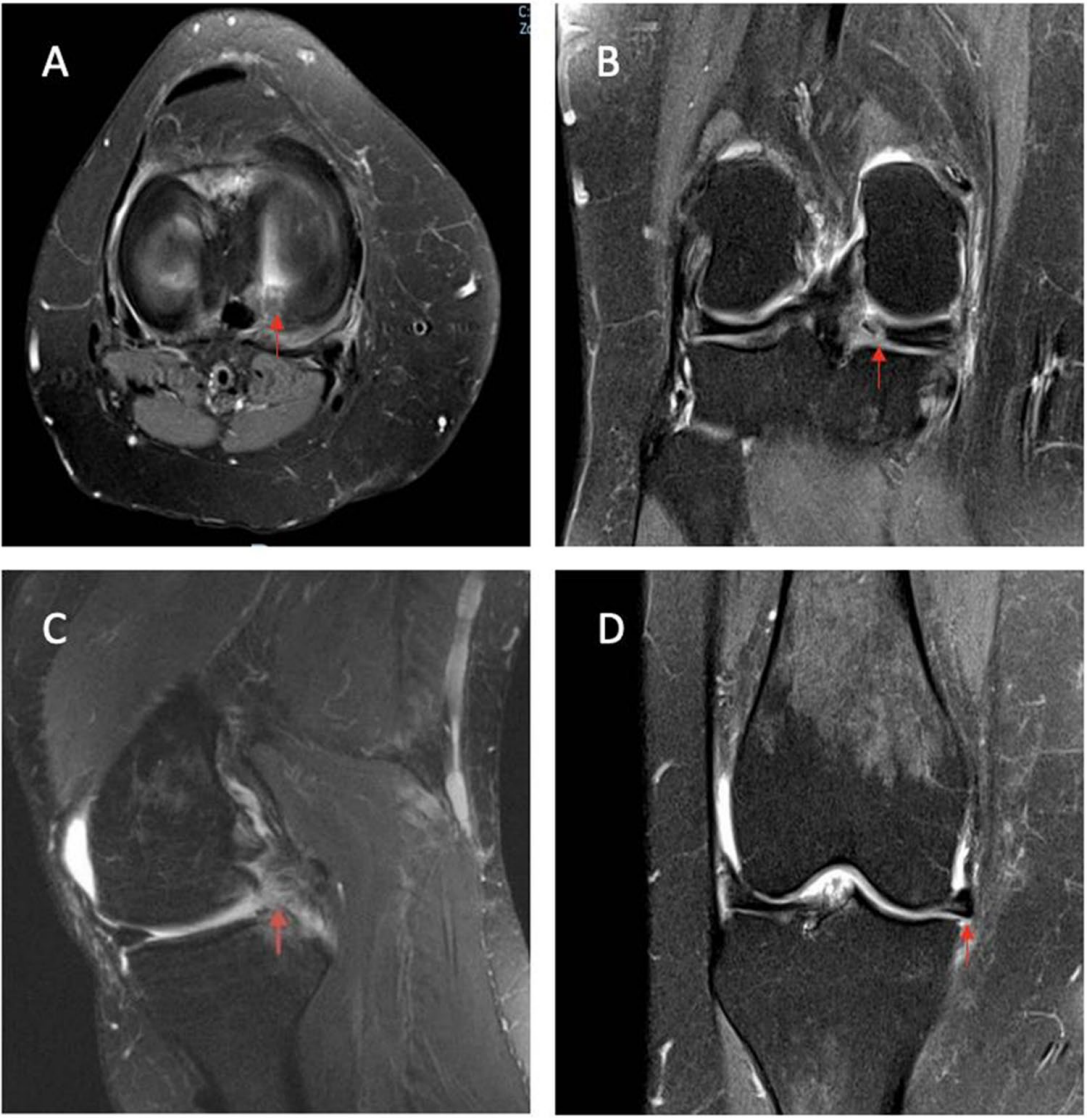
Direct and indirect MRI signs of meniscal root tears. T2 MRI images showing A high signal in the region of meniscus root and posterior horn on axial images; B Cleft sign; C Ghost sign and subchondral edema on sagittal image; and D meniscus extrusion on coronal image
